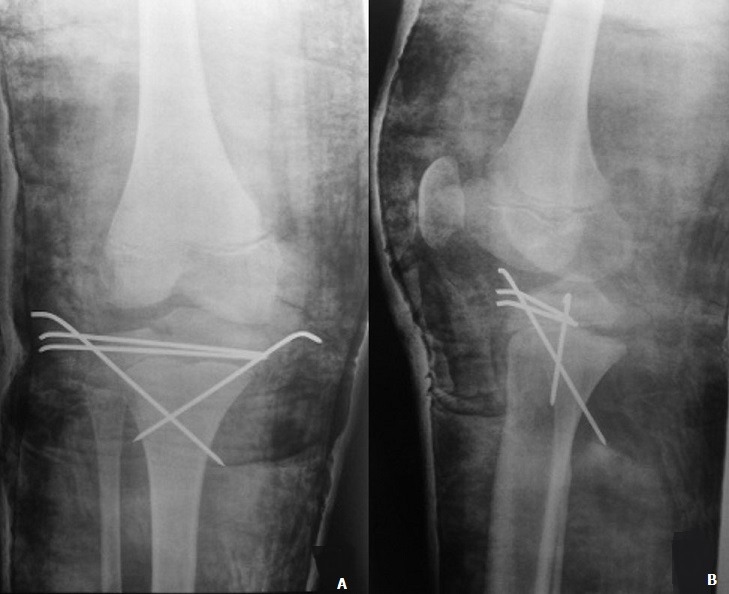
(A) post-operative anteroposterior and lateral (B) radiographs of the right knee
